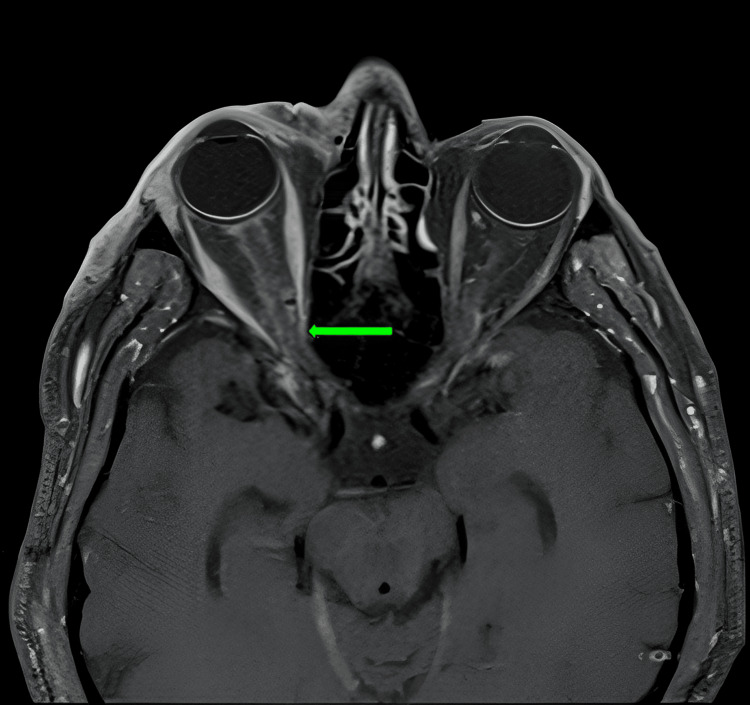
An axial MRI image with contrast demonstrating enhancement of the perineural sheath of the optic nerve in the right eye (green arrow).
